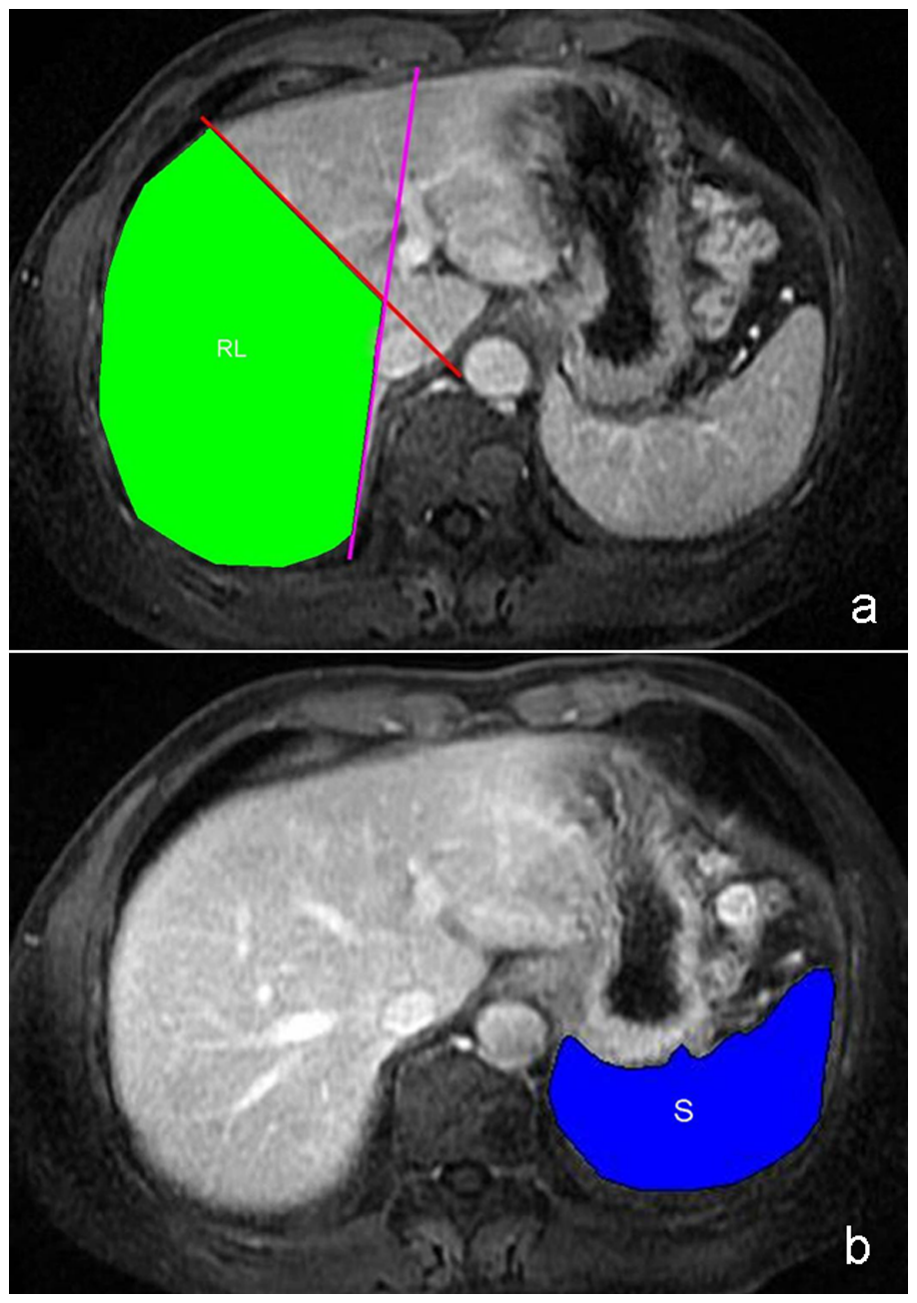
The outline of right liver lobe. Middle liver vein (red line) is used as a landmark to differentiate right liver lobe from left liver lobe, and the line linking the inferior vena cava to the right branch of the portal vein (pink line) is used as a landmark to differentiate right liver lobe from caudate lobe (a). Outline of right liver lobe (RL, a), and of spleen (S, b) are delineated on the axial enhanced magnetic resonance imaging.
